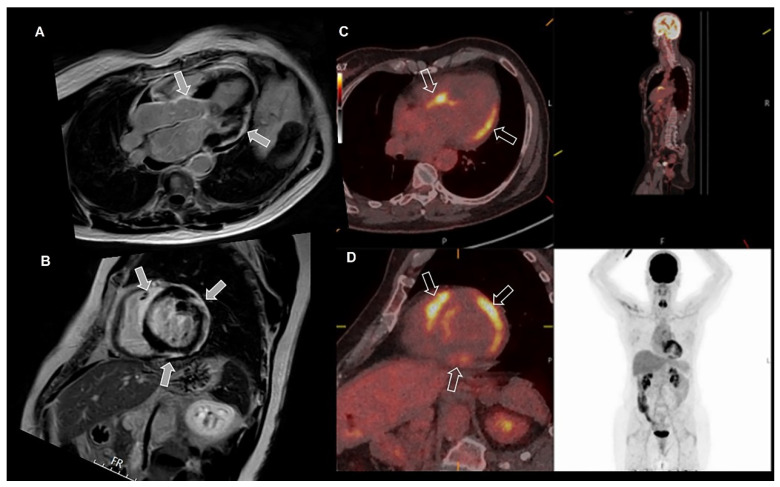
Cardiac sarcoidosis diagnosed with cardiac magnetic resonance (CMR), positron emission tomography (PET) and computed tomography (CT). The figure shows the presence of subepicardial late gadolinium enhancement inferior and lateral, and midmyocardial in the basal interventricular septum (arrows), suspicious for sarcoidosis on CMR (A,B). PET-CT images (C,D) show corresponding increased uptake (arrows) of fluorodeoxyglucose, indicating inflammatory lesions associated with sarcoid activity in the corresponding areas, enabling the diagnosis of sarcoidosis. Note the absence of tracer uptake outside the heart indicating isolated cardiac sarcoidosis.
